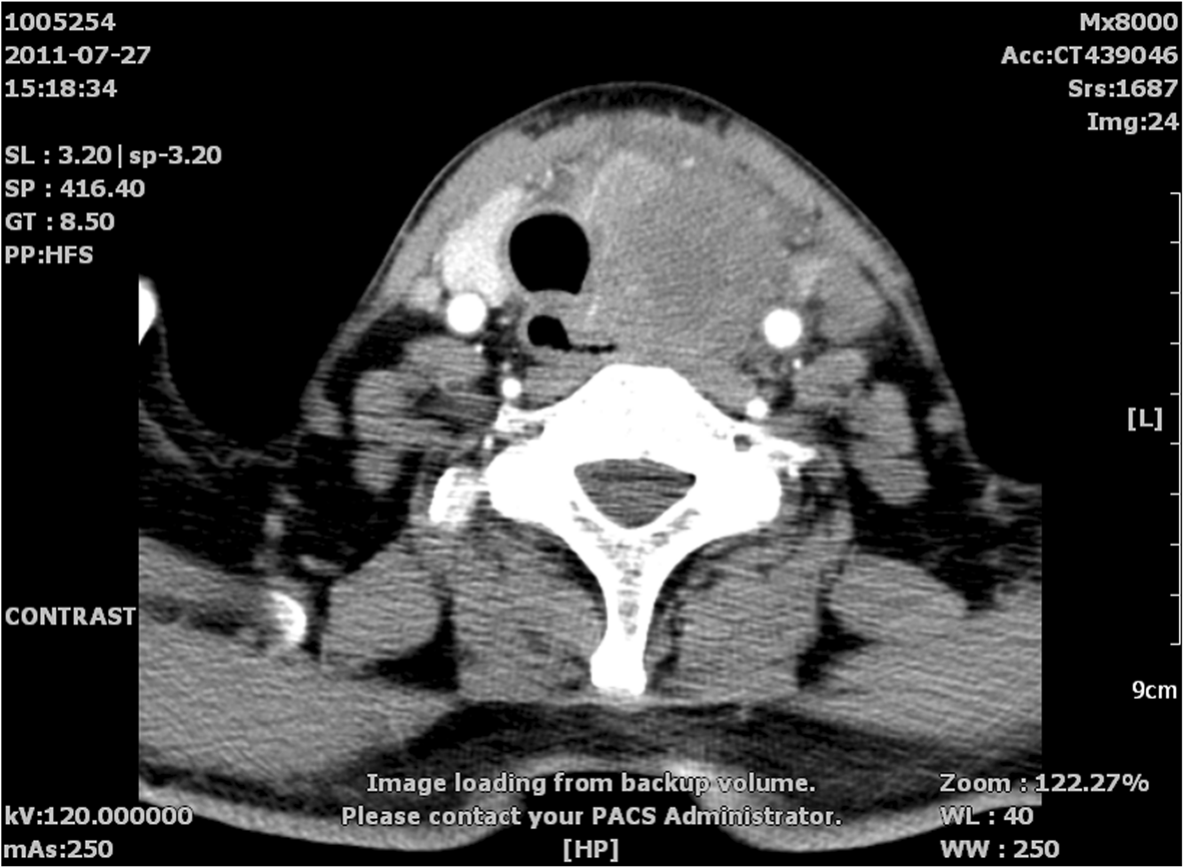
Neck contrast-enhanced computed tomography reveals a mass encasing the left carotid sheath vessels, esophagus, and trachea in the left thyroid lobe.
